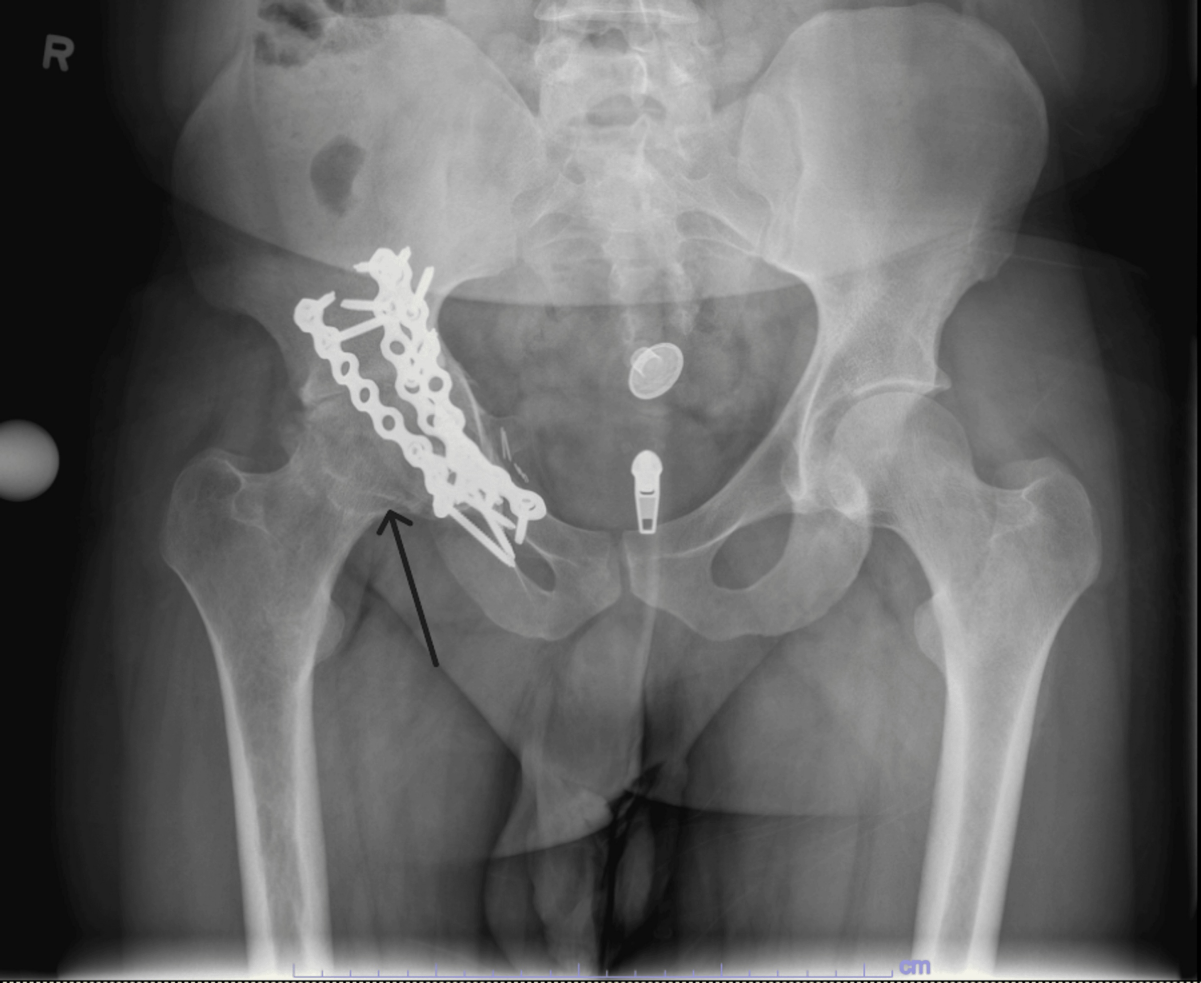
Preoperative AP X-ray of the pelvis demonstrating a femoral neck fracture with acetabular plating Arrow indicates the femoral neck fracture. AP, anteroposterior
